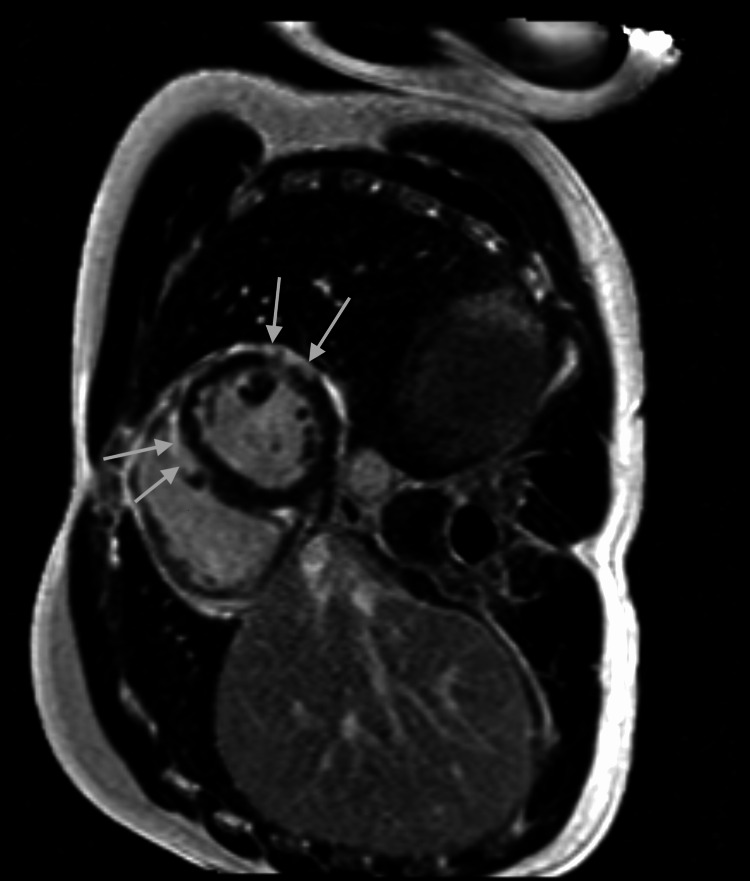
Cardiac MRI showing diffuse subepicardial enhancement of the left ventricular walls with relative sparing of the base, consistent with myocarditis Short-axis cardiac MRI performed at 1.5 T shows myocarditis. The delayed contrast-enhanced image shows diffuse subepicardial enhancement of the left ventricular walls (arrows) with relative sparing of the base.
